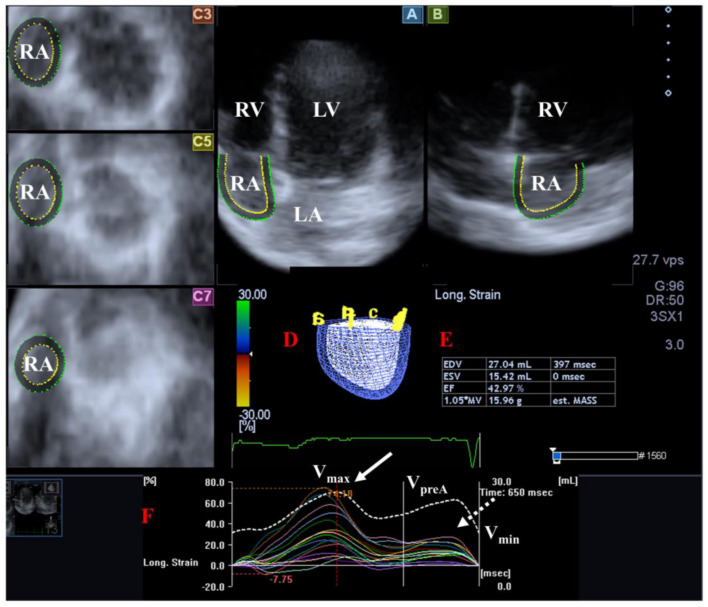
Examination of the right atrium (RA) by three-dimensional (3D) speckle-tracking echocardiography. Following echocardiographic data acquisitions, the following typical views are created: (A) Apical 4-chamber and (B) two-chamber longitudinal views and (C3,C5,C7) cross-sectional views at basal, midatrial and superior levels, respectively. RA can be easily detected alongside other heart cavities including the left ventricle (LV) and atrium (LA) and the right ventricle (RV). A number of other details were also presented including (D) 3D cast and (E,F) maximum (V_max_), preatrial contraction (V_preA_) and minimum (V_min_) volumes of the RA and curves representing changes in volumes and strains of _RA over time. Reservoir (peak) and active contraction RA strains are represented by white and dashed white arrows, respectively.
